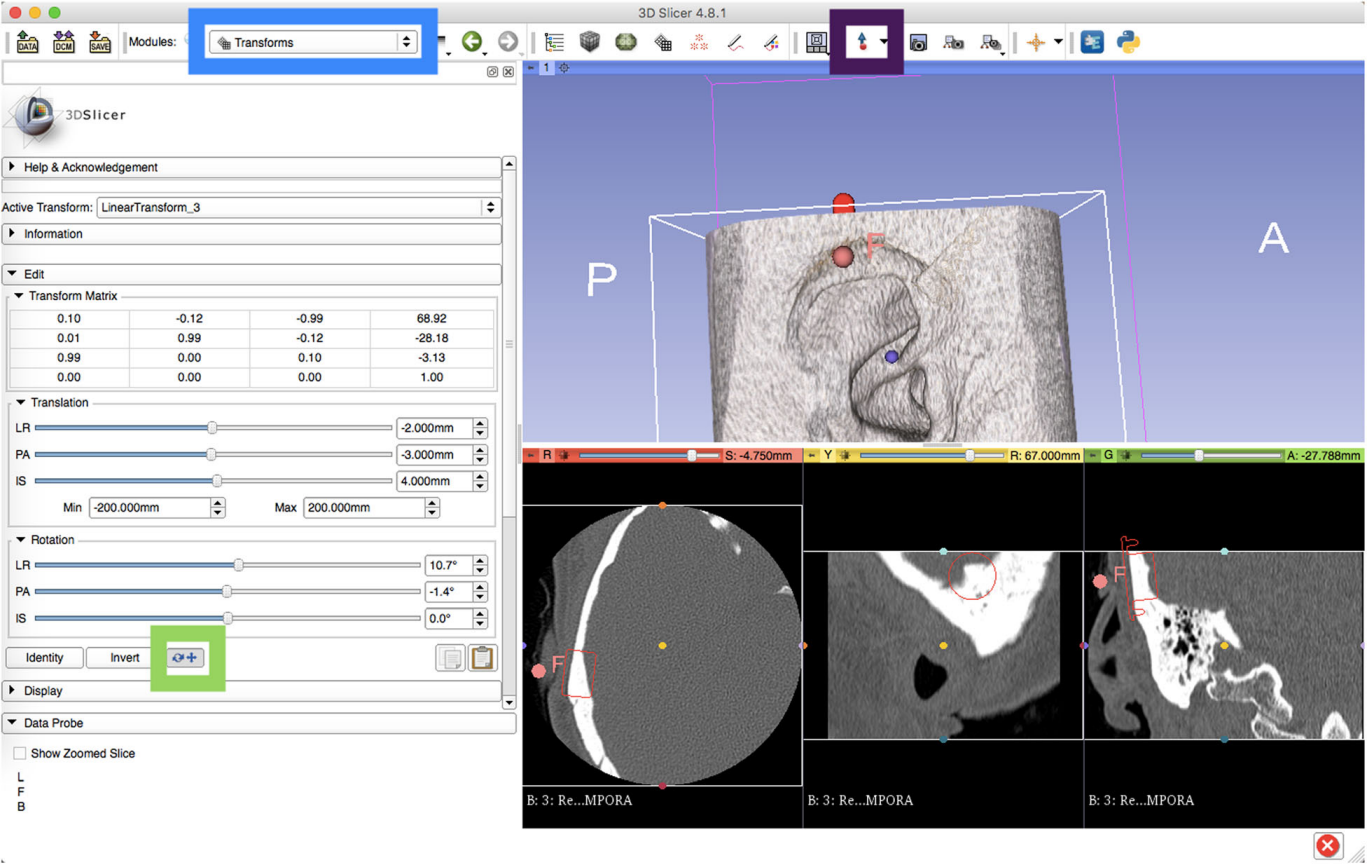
Screen capture of the 3D Slicer interface in Conventional view for preoperative planning of middle fossa approach to BONEBRIDGE implantation. Traditional axial, sagittal, and coronal views of a temporal bone CT are visible at the bottom of the screen. The BC-FMT is highlighted with a red outline on these slices, and a red fiducial marks the centre of the implant on the skin. Blue box = module drop down menu. Green box = option to select Transform selection to alternate between global versus regional transform. Purple box = option to add fiducials. F = digital fiducial
